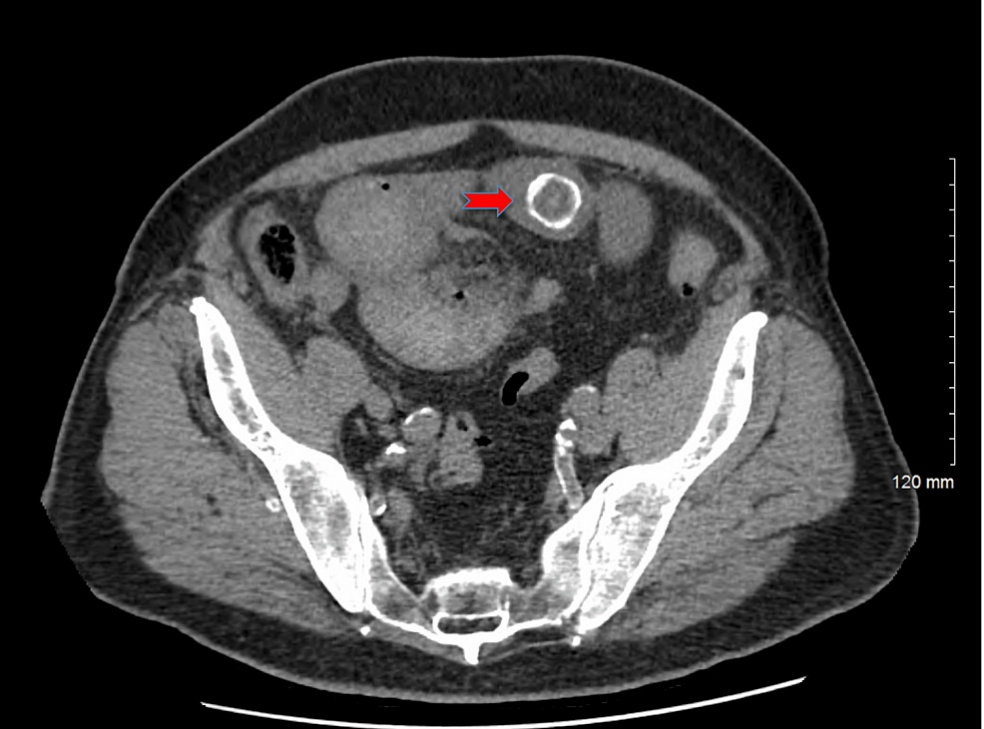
Axial CT highlighting an impacted gallstone in jejunum (red notched arrow).
